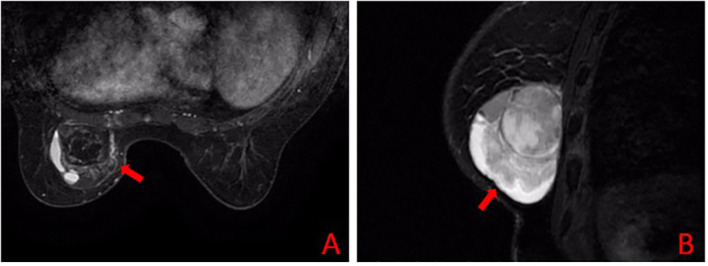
Preoperative breast MRI. (A) axial and (B) sagittal contrast-enhanced T1-weighted images show a large, lobulated, heterogeneously enhancing mass (arrows) in the left breast.
